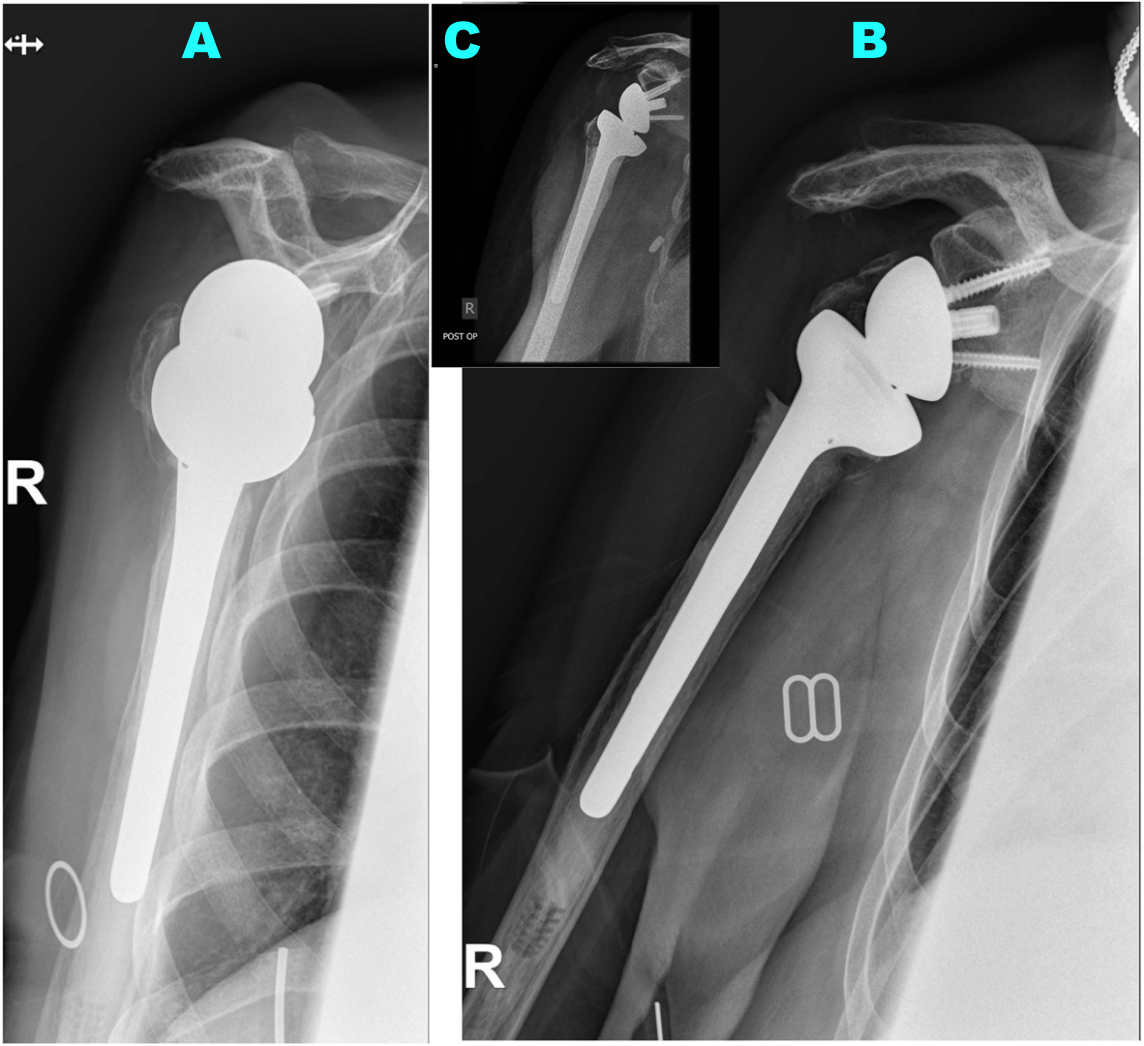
Malunion of the greater tuberosity: A case of a 78 years old woman, a X-ray is pictured in three planes 35 months post operation. A post operation index X-ray (c) is added for comparision of the greater tubercle to final radiological outcome. (a: supraspinatus outlet view, b: anterior-posterior view, c: anterior-posterior view in X-ray two days post operation)
